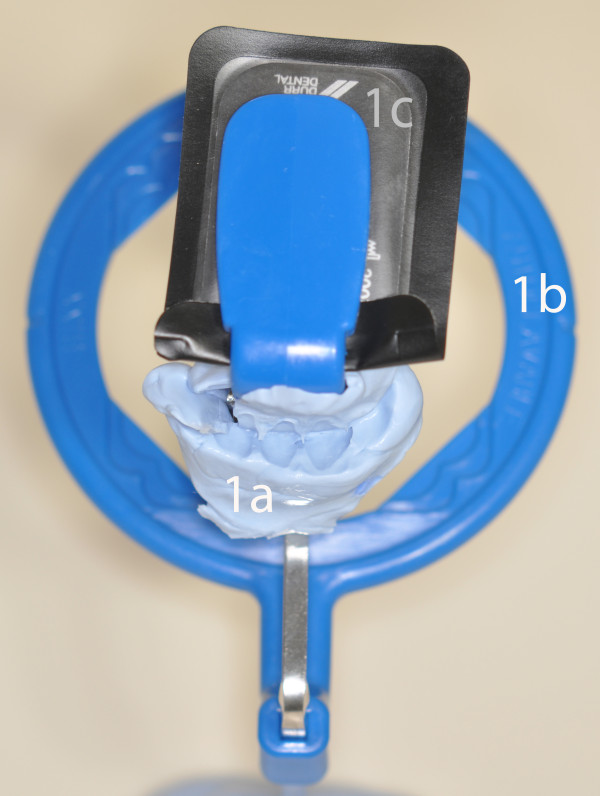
Device used to realize reproducible radiographs at each session. The patient is asked to bite into a piece of silicone, which enables us to put the film holder and so the radiograph in the same position at each session. 1a: piece of silicone. 1b: film holder. 1c: Radiograph.
